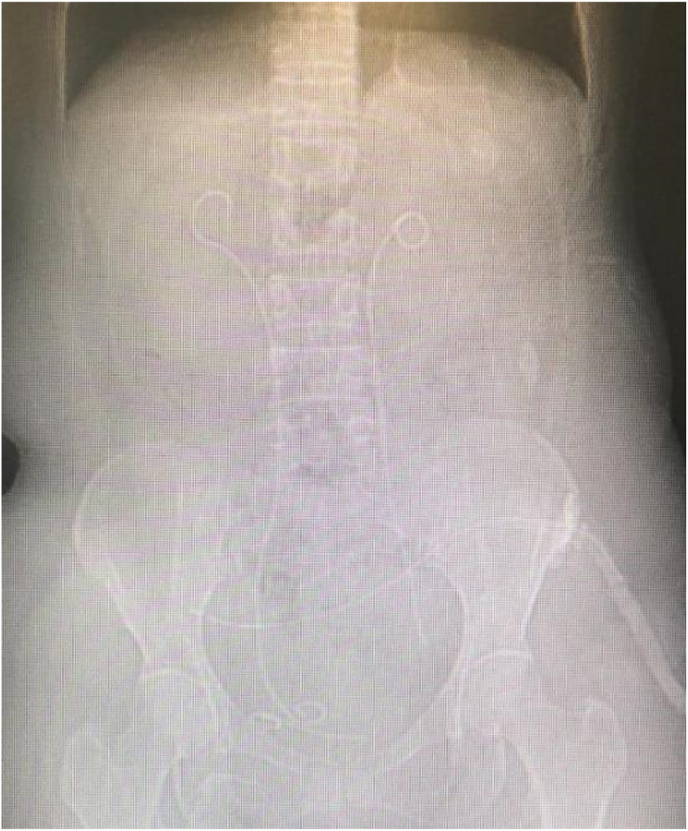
Plain KUB showed left broken Double-J. KUB, kidney, ureter, and bladder radiograph.
